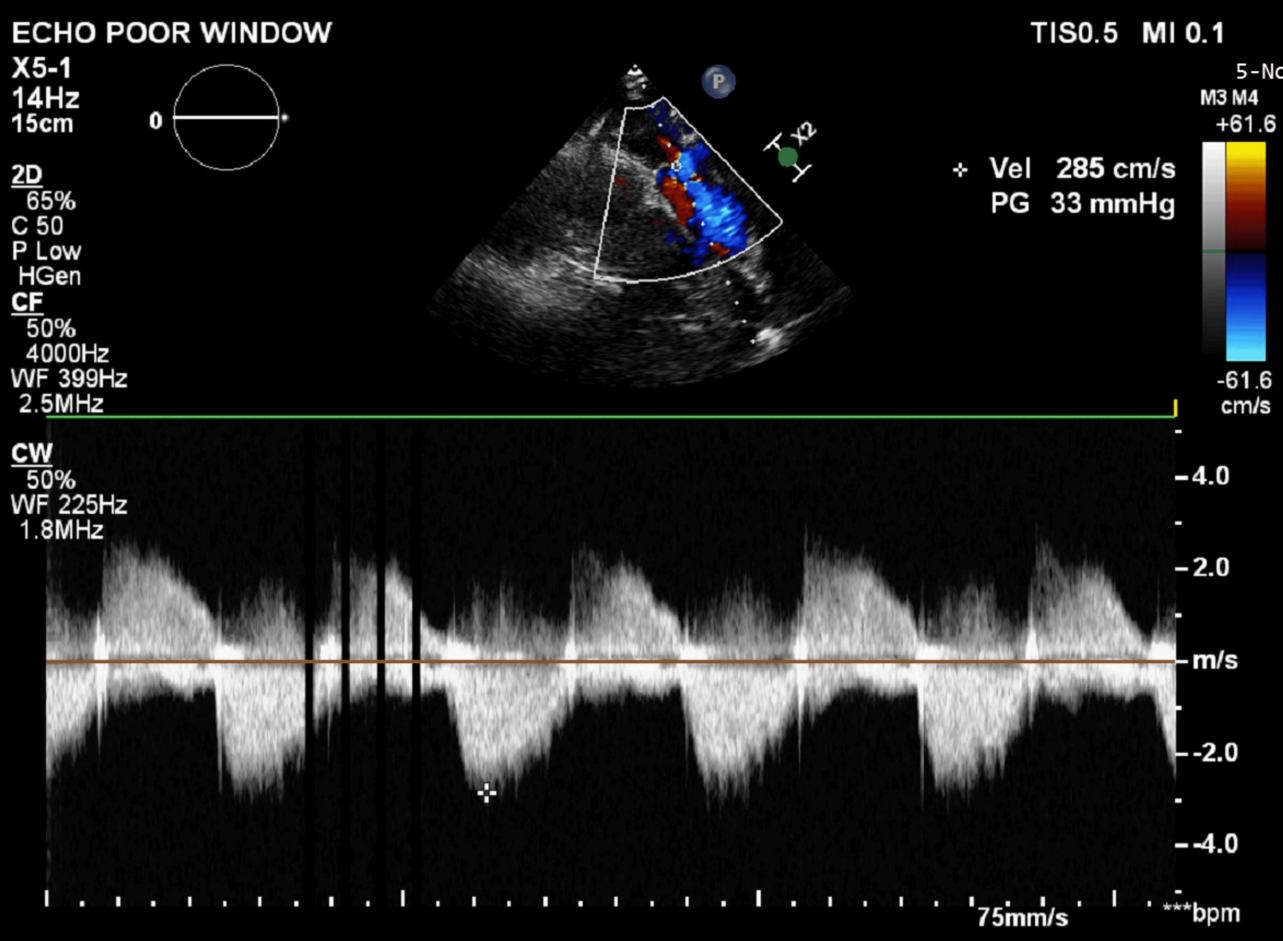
Follow-up echocardiography (PLAX RV outflow view) showing a pulmonary valve gradient of 33 mmHg with mild pulmonary regurgitation. PLAX: parasternal long-axis; RV: right ventricular
